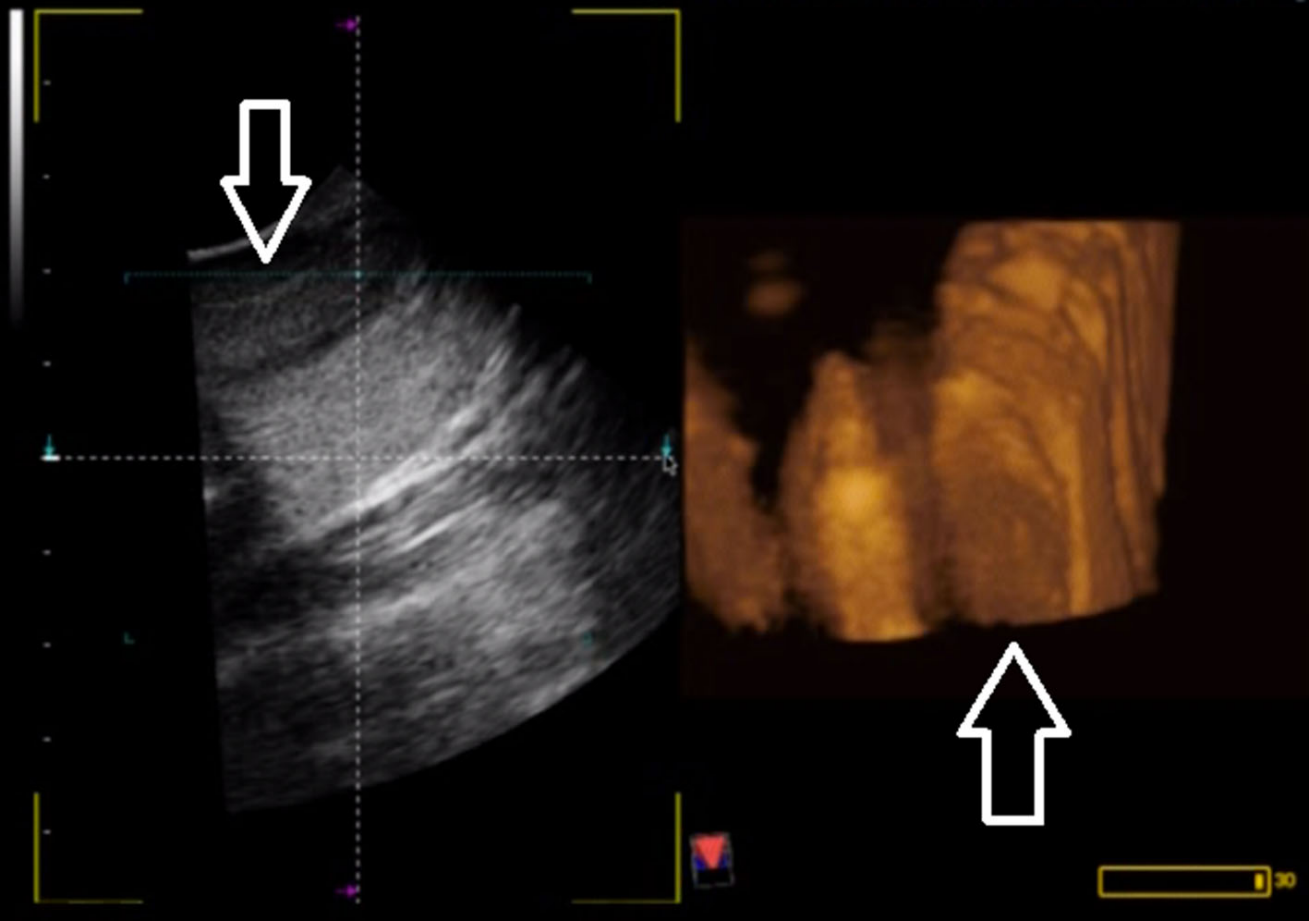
Incidental nodule found during ultrasound training. 2D ultrasound was used initially, as the thyroid nodule was discovered (*left*). Imaging using 3D/4D ultrasound (*right*) depicts the nodule and allows to see the nodule with both height and width from this viewpoint. The 3D/4D image can be rotated to further investigate the depth of the nodule, allowing for a better understanding of the size and shape of the nodule
